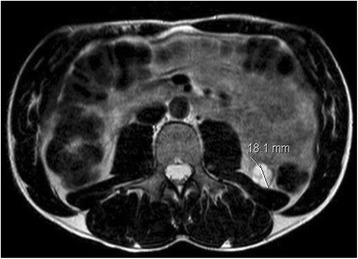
Abdominal MRI showing a 2 × 2 × 1.8 cm exophytic lesion in the lower pole of the left kidney demonstrating septation and enhancement
